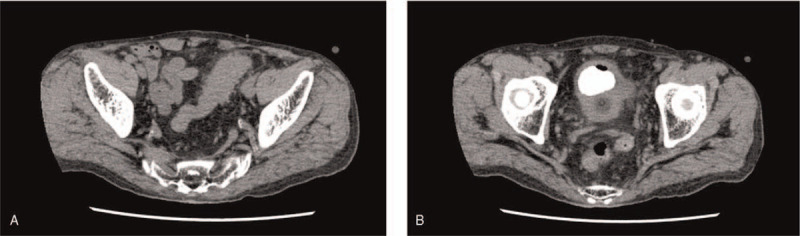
The representative images of abdominal computed tomography (CT). (A) Abdominal computed tomography findings show multiple sigmoid colon diverticula with thickened walls. (B) Large stones and a small amount of air are found in the bladder, suggesting the presence of a colonic cystic fistula.
